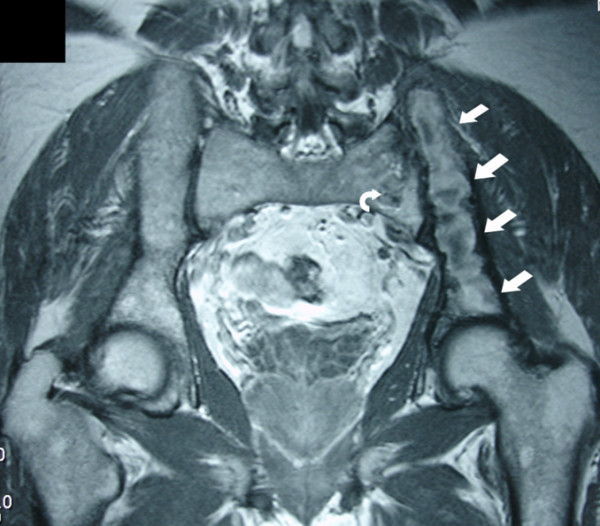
Post contrast coronal T1-weighted MRI shows a heterogeneous increased signal at the left iliac bone (arrows) and at the left sacral wing.
